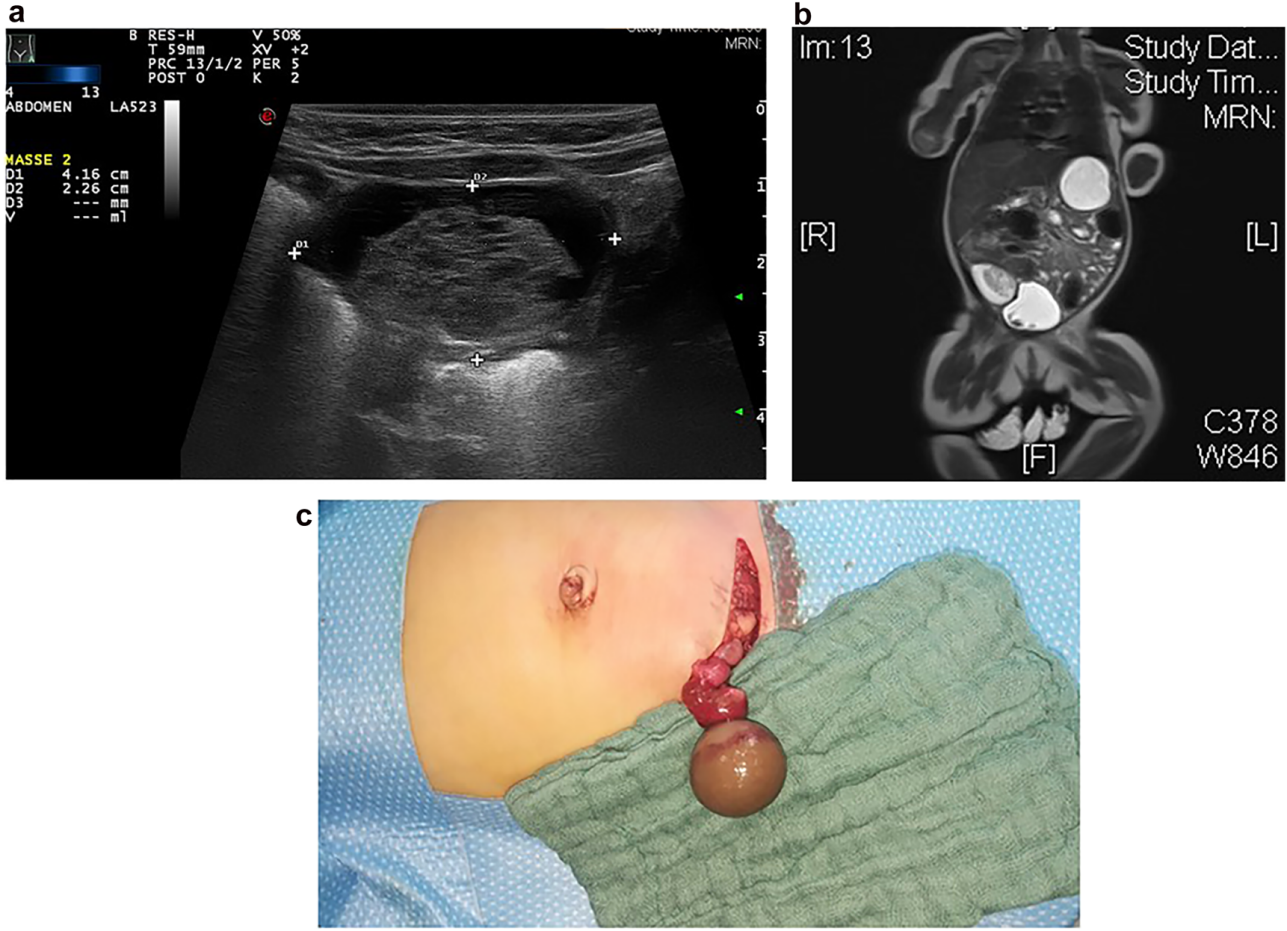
Sonography (a) and MRI (b) findings showed a cystic mass in a 6 week old infant with hemorrhagic solid mass and fibrous septation of the cystic mass typically suggesting ovarian necrosis. Intraoperative situs (c) revealed an old (intrauterine) ovarian torsion with a necrotic ovary.
